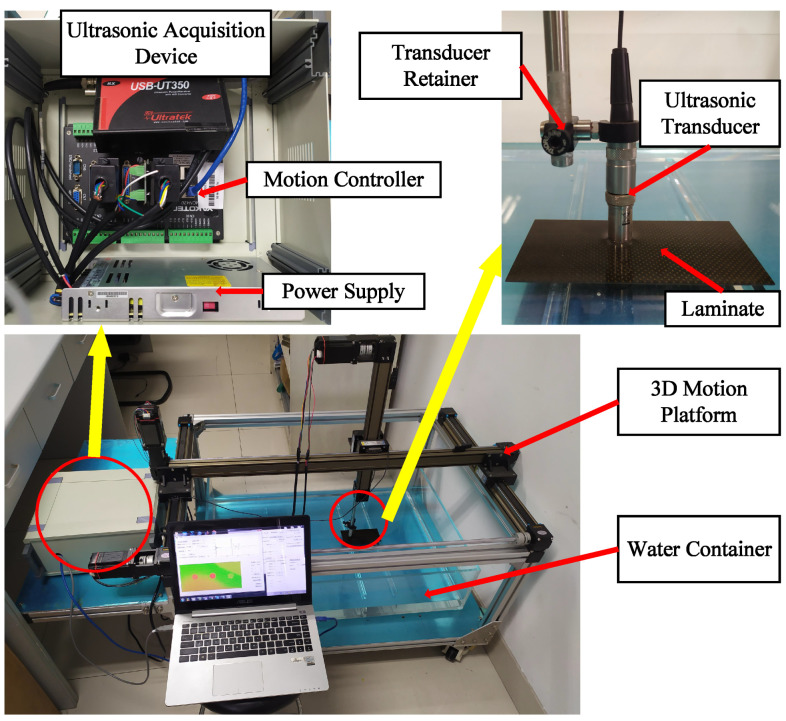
Ultrasonic immersion scanning system.
